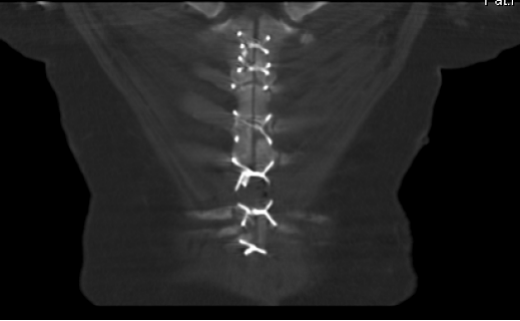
A computed tomography (CT) scan prior to supportive therapy showing sternal non-union (two months after operative refixation).
